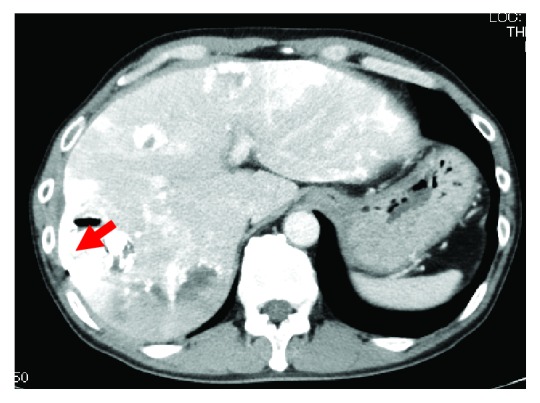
CT scan showing the liver after TACE and HAIC. The red arrow indicates the site of the ruptured mass.
